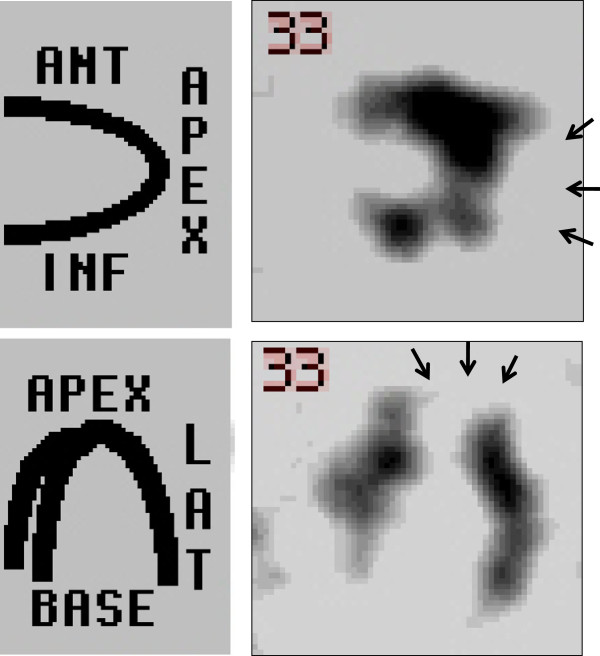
Technetium-99m tetrofosmin single-photon emission computed tomography scan demonstrates an area of severe hypoperfusion at the left ventricular apex (arrows).
